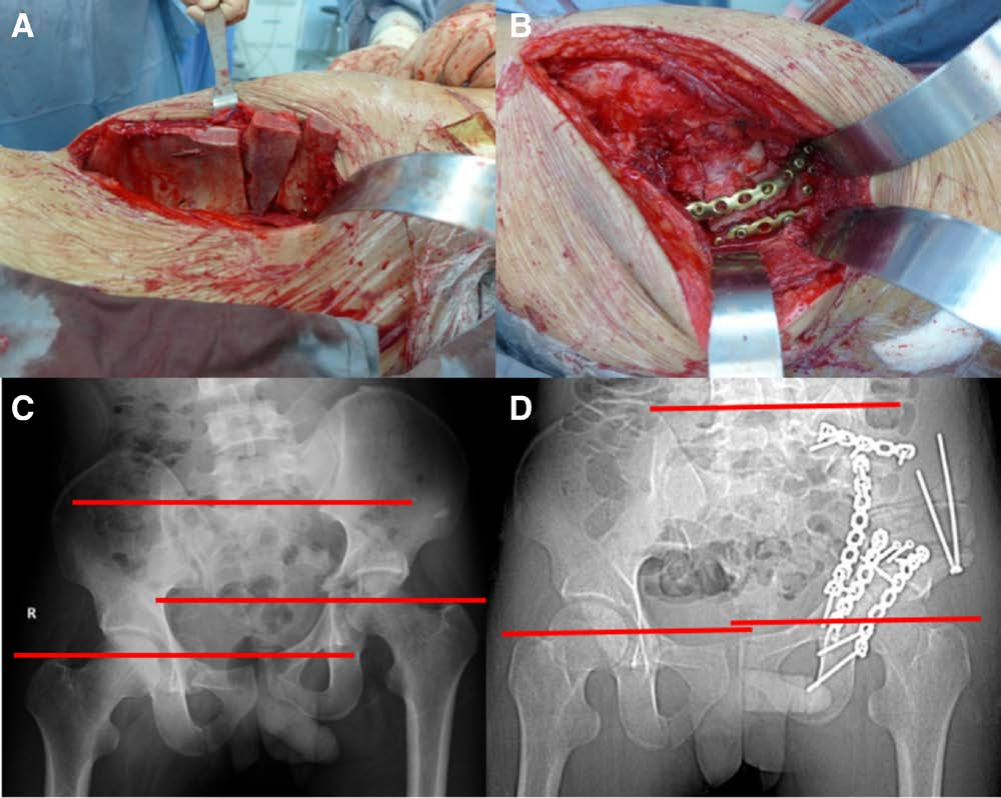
(A) Intraoperative bone graft after osteotomy. (B) Fixation with pre-bent plates. (C) Preoperative image showing limb discrepancy. (D) Postoperative radiography showing correction of limb discrepancy. (C and D) Originates from our previous study^[30]^ and was authorized by the Chinese Journal of Orthopaedics.
